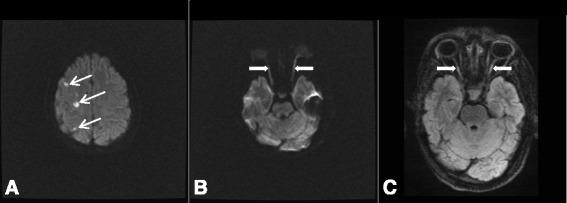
Magnetic resonance imaging of patient’s brain. a Axial diffusion-weighted image demonstrating small strokes to the *right* frontal and parietal lobes (*white arrows*). b and c are axial diffusion-weighted image and fluid-attenuated inversion recovery image demonstrating bilateral optic nerves thickening and increased signal compatible with ischemic injury (*white arrows*)
